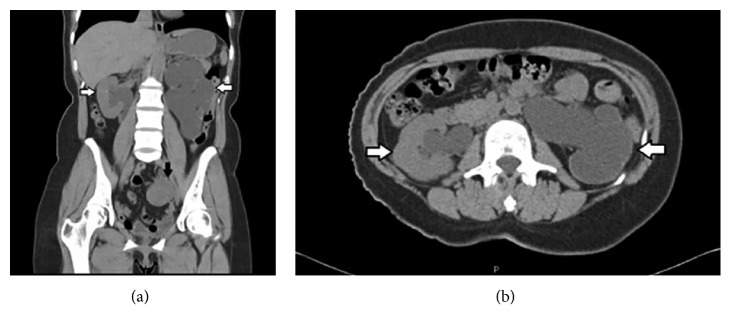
(a) Computed tomography showing a 4.5 cm sized left endometriotic cyst (black arrow) causing ureteral obstruction with severe left hydroureteronephrosis. Enlarged kidneys, with complete loss of left renal parenchyma (white arrows). (b) Milder right hydroureteronephrosis (white arrows) [110].
